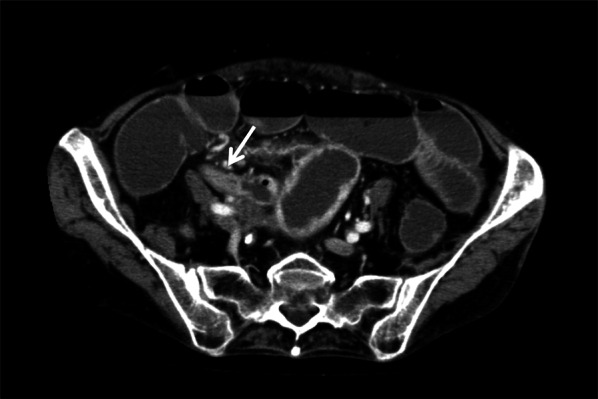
Computed tomography demonstrates that the whole bowel is distended, with an air-fluid level in the small intestine. Wall thickening and stenosis of the terminal ileum (white arrow) are found
